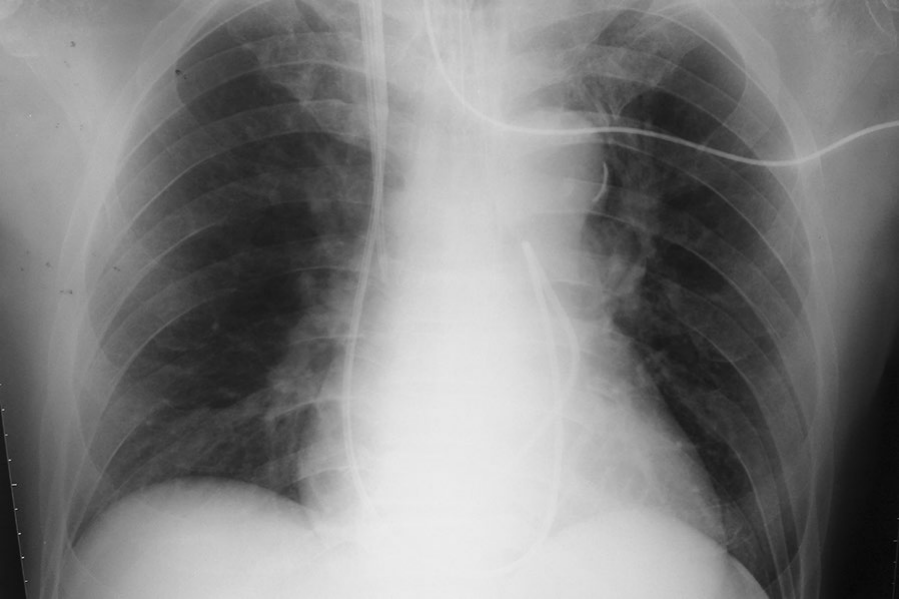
Chest X-ray showing kinked pulmonary catheter
